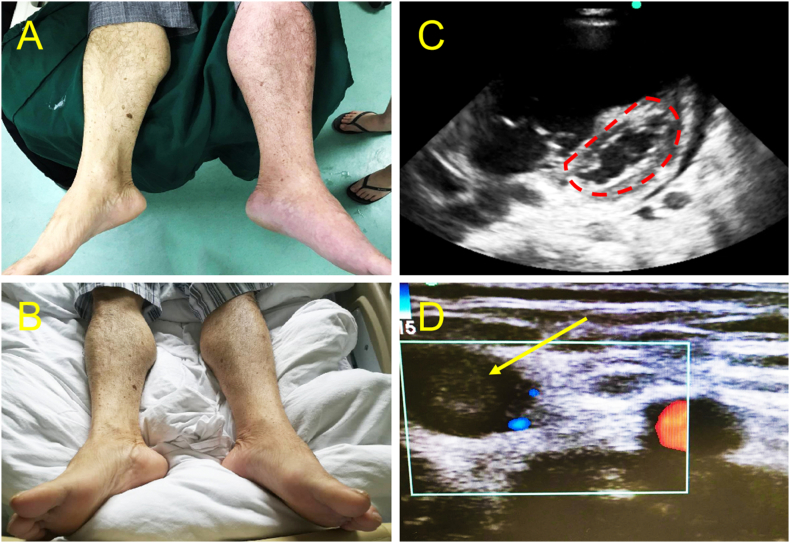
Lower extremity point-of-care ultrasound of patient 3. A: Cyanosis noted on left lower limb before thrombolytic therapy; B: improvement of discoloration on left lower limb after thrombolytic therapy, C: parasternal short axis shows D shape sign; D: visible thrombosis noted in left femoral veins.
